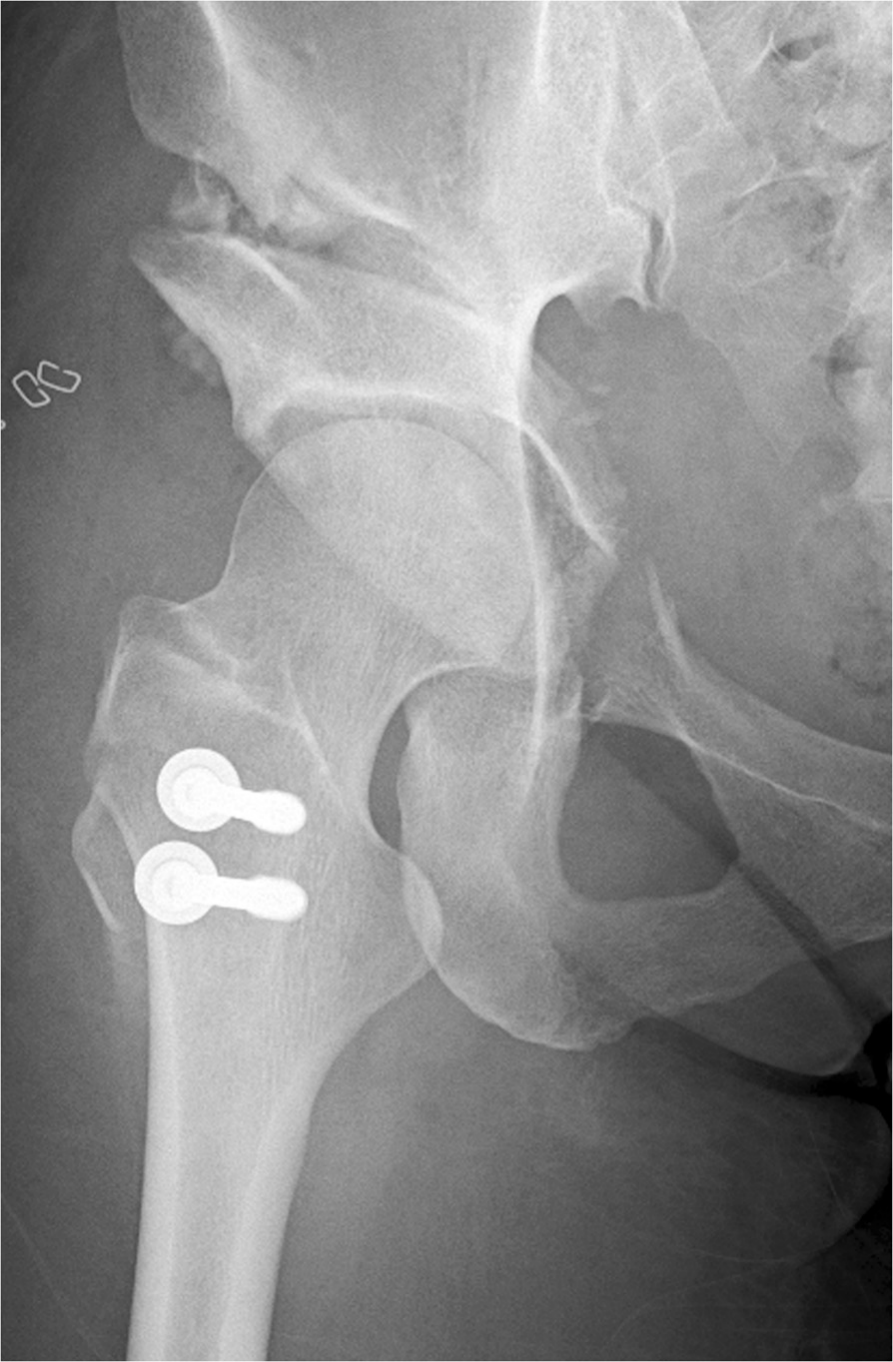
A radiograph of the same patient made 1 week after periacetabular osteotomy showing good coverage of the femoral head without fracture of the posterior column. The accelerated rehabilitation protocol was applied to the patient.
